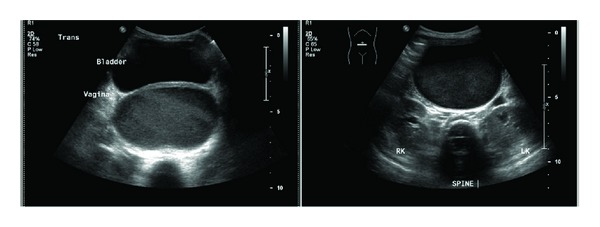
Pelvic ultrasound of Neonate A showing fluid collection in both bladder and vagina.
